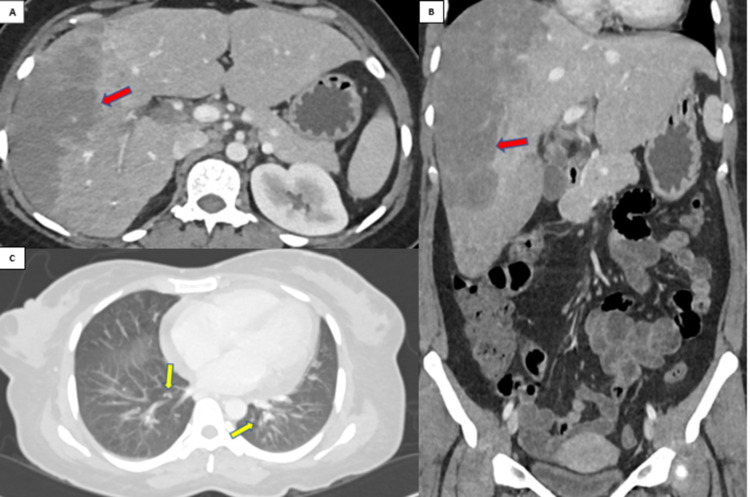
CT abdomen and pelvis with contrast, (A) axial and (B) coronal portal phase, showing enlarged liver with large ill-defined hypodense area in the right lobe (red arrows). (C) Axial lung window showing bilateral lung nodules.
